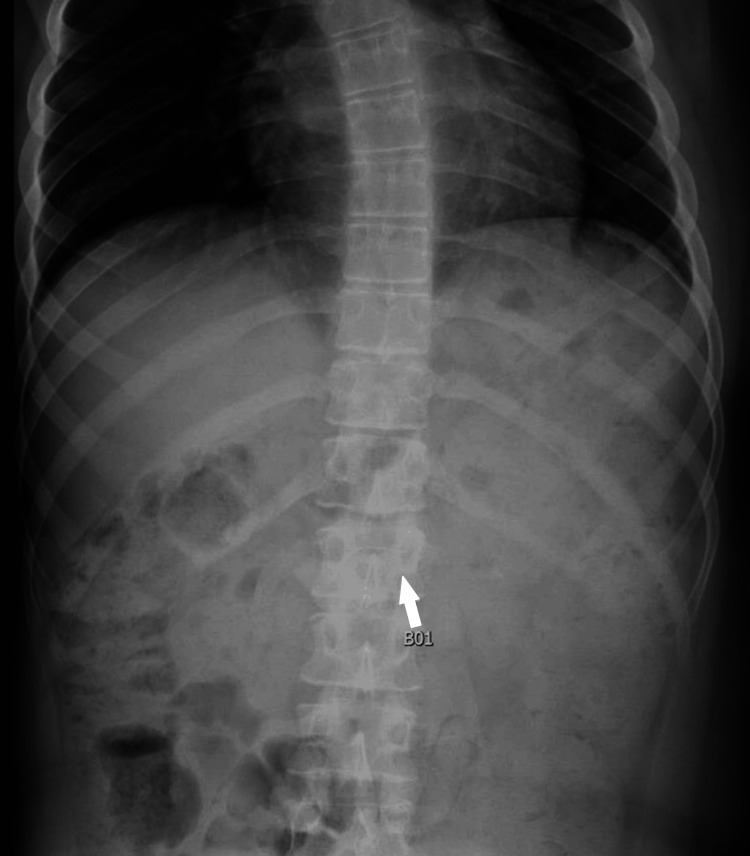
Initial abdominal radiograph obtained to evaluate gastrostomy tube placement. Anteroposterior and lateral views demonstrating the gastrostomy tube in situ but without clear visualization of the distal tip within the gastric lumen. The white arrow indicates a vertebral body used as an anatomic reference point, emphasizing that the gastrostomy tube tip is not definitively identified on the radiograph, rendering the imaging nondiagnostic.
